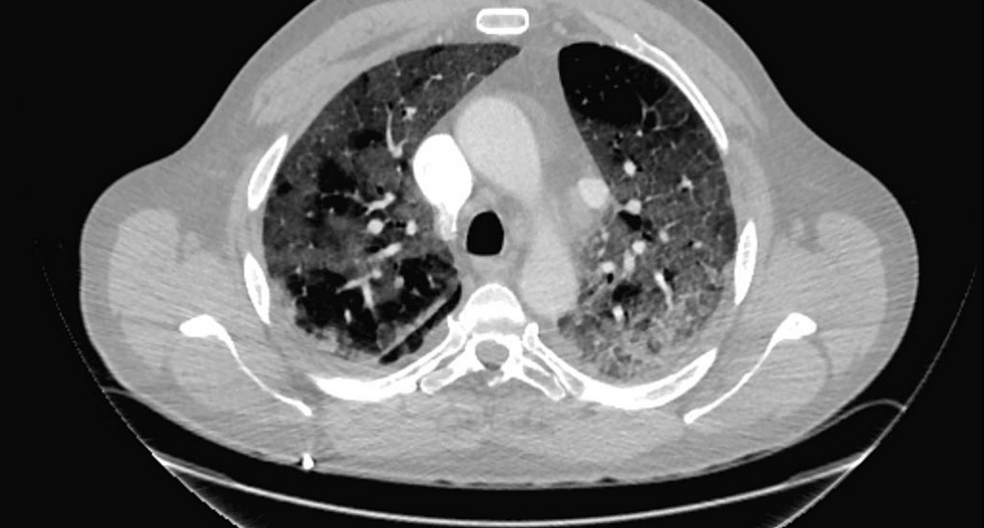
CT scan of the chest on day 7 of admission
